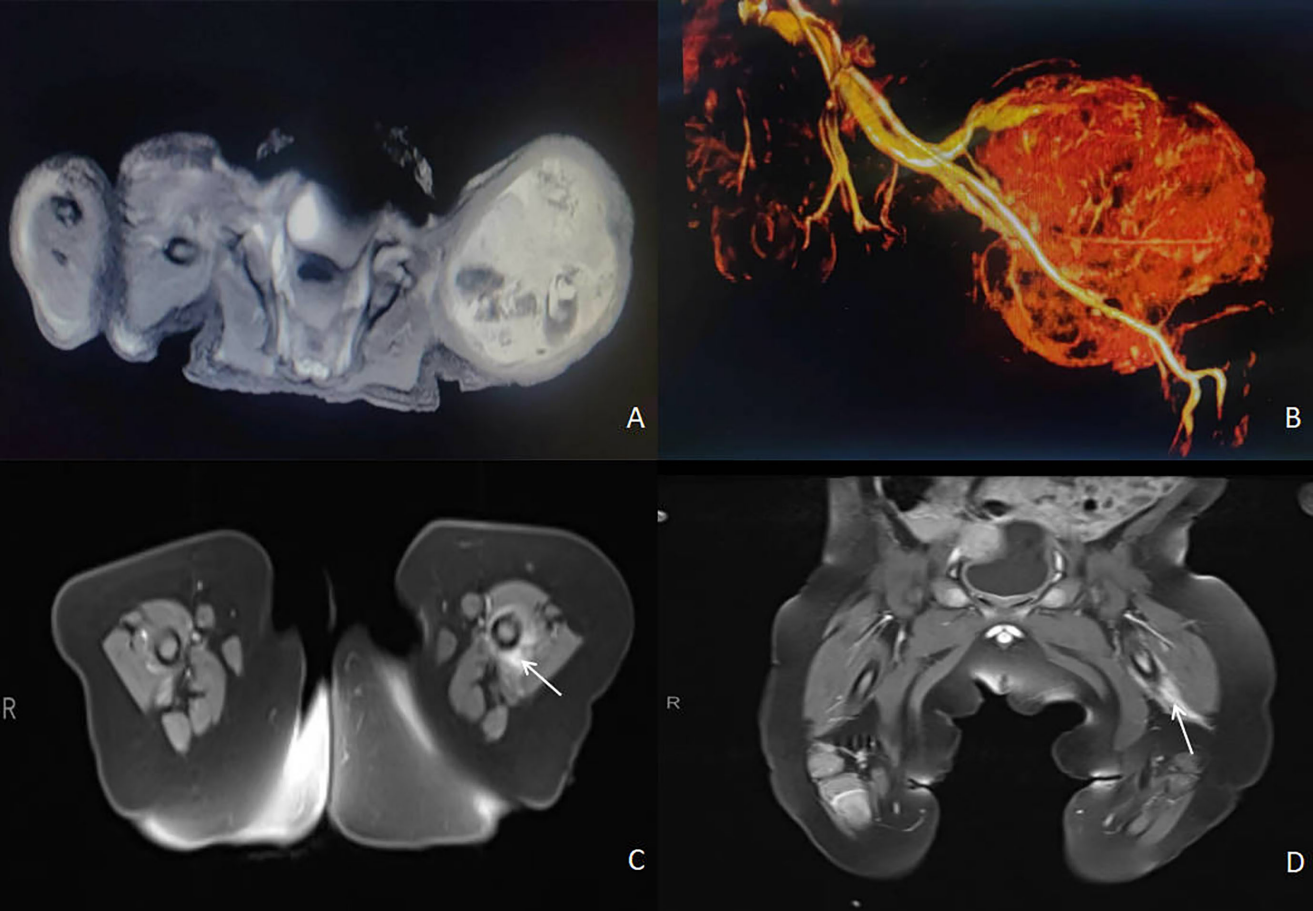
Imaging results. (A) MRI examination of the lower limb: a circular soft tissue mass in the left lower limb, measuring 8.25cm x 8.72cm x 7.86cm, closely adjacent to the femur and surrounding it, with unclear demarcation from the surrounding muscle tissue, and mixed-signal intensity in the mass. (B) enhanced CT of the lower limb: the mass is mainly supplied by the left femoral artery, with flux into the femoral vein, and many tortuous vessels are visible within the lesion. (C, D) MRI of the lower extremity revealed a significant mass reduction after four months of treatment.
